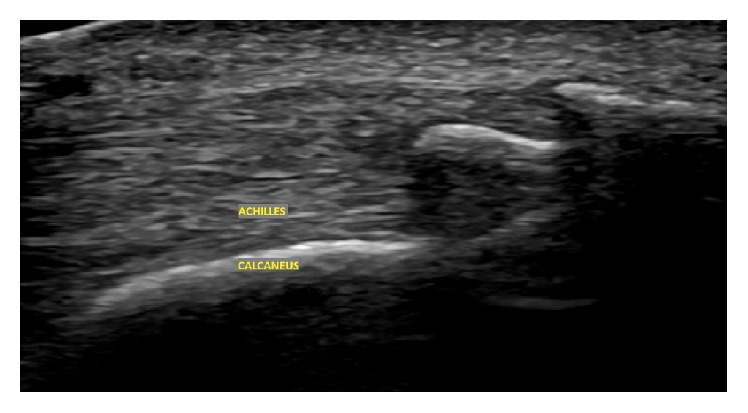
Long axis view ultrasound evaluation in a male with long-time chronic symptoms shows enthesophyte irregularity of the cortex of the calcaneus as well as intratendinous calcification at the Achilles tendon insertion.
